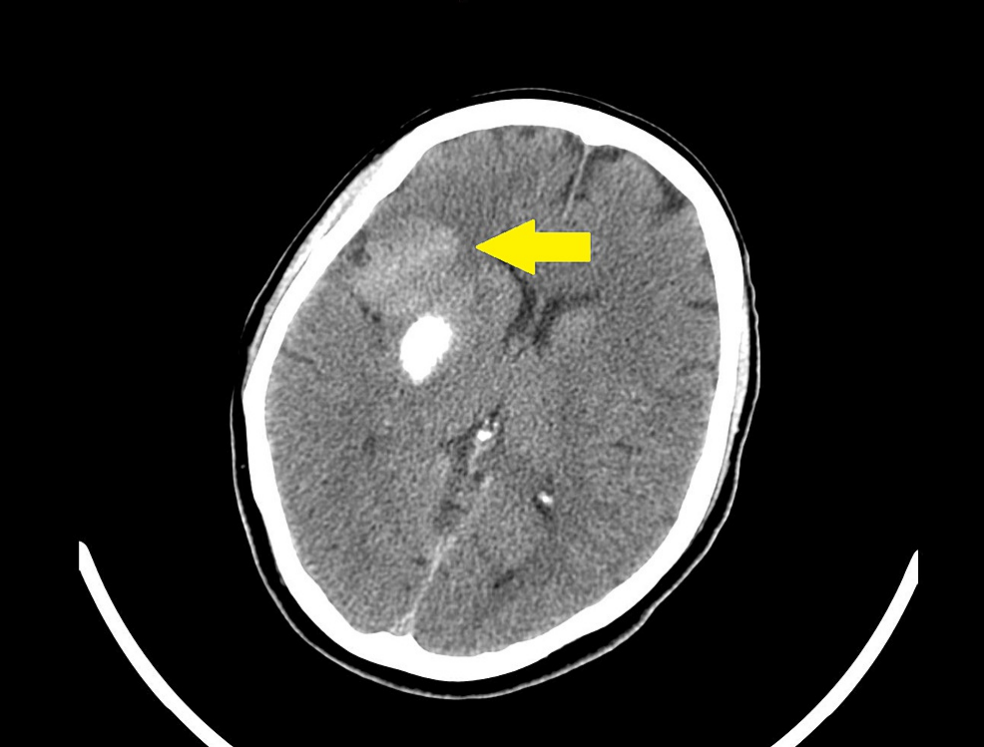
CT of the head showing hemorrhagic conversion in the basal ganglia (yellow arrow). CT: computed tomography.
